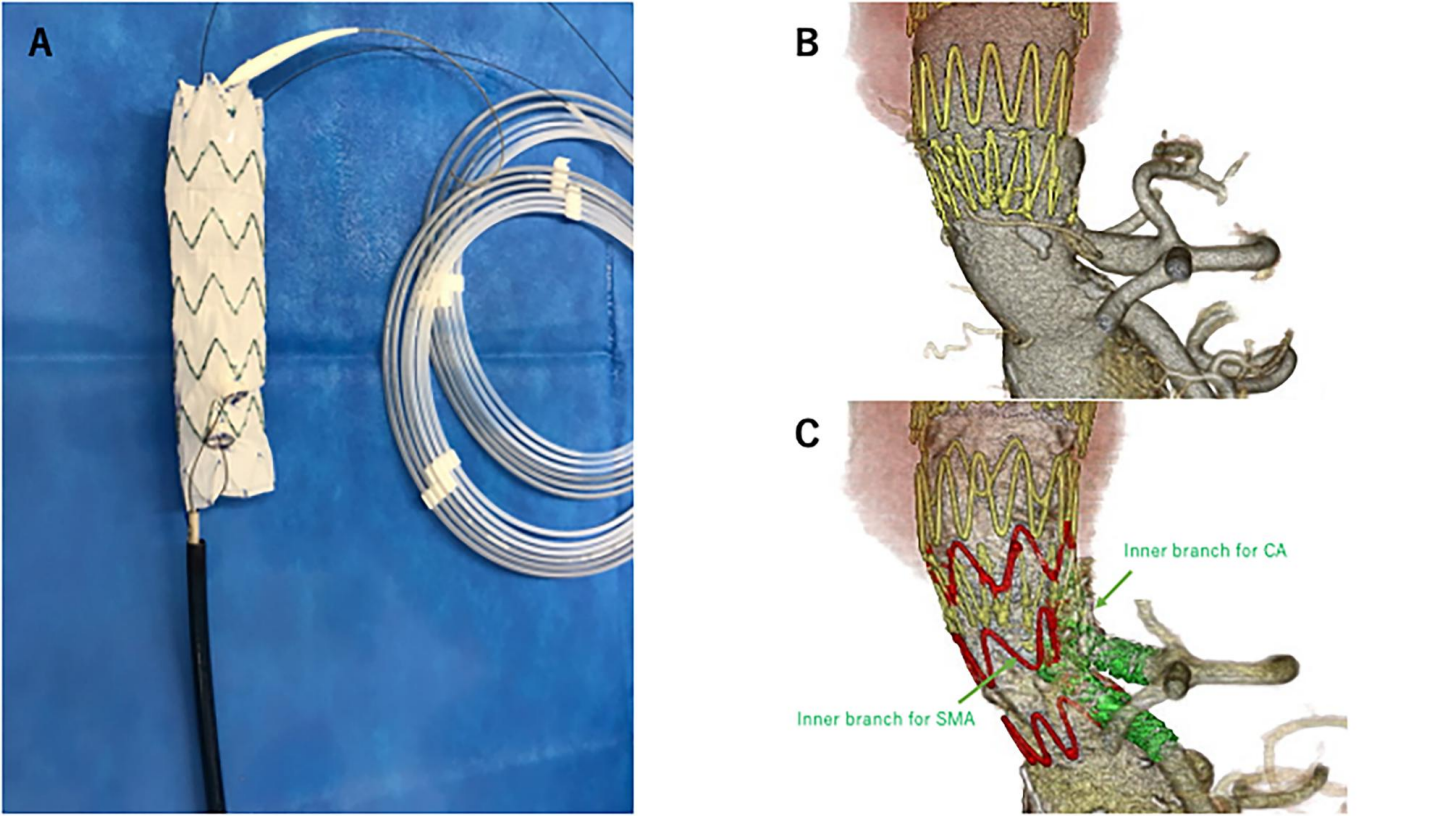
An 82-year-old man with an enlarged thoraco-abdominal aortic aneurysm due to a type Ib endoleak underwent a physician-modified endovascular graft with inner branches. (A) The physician-modified endovascular graft with inner branches for coeliac artery and superior mesenteric artery Zenith alpha thoracic stent graft back-table preparation. (B) Preoperative computed tomography scan. (C) Postoperative computed tomography scan. CA: celiac artery; SMA: superior mesenteric artery.
